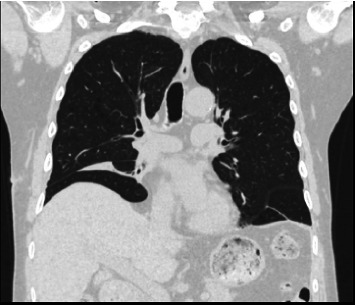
Chest CT scan following evacuation thoracentesis.
